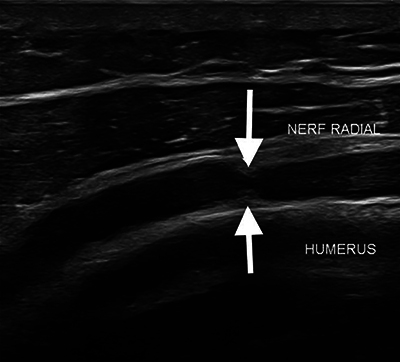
Ultrasound shows focal central narrowing of the radial nerve at the site of its posterior humeral course, with diffuse hypoechoic thickening both upstream and downstream (arrows).
